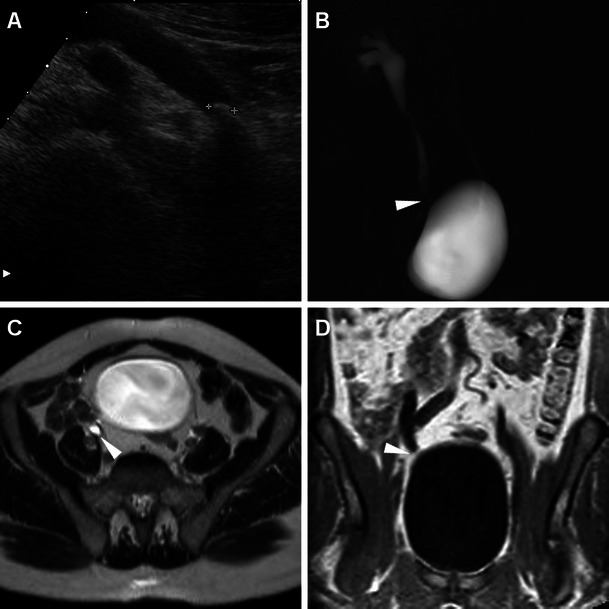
A 51-year-old male received ureteroscopic removal of a distal ureteral calculus (*ultrasound in A*). Fifteen months later, persistent upstream hydronephrosis without evidence of lithiasis at unenhanced MDCT (not shown) led to performing unenhanced magnetic resonance (MR) urography. Pyelographic (B) and axial T2-weighted images showed smooth tapering of the pelvic ureter (*arrowheads*), without appreciable mural thickening or extrinsic tissue at the coronal T1-weighted image (D), consistent with an iatrogenic stricture
